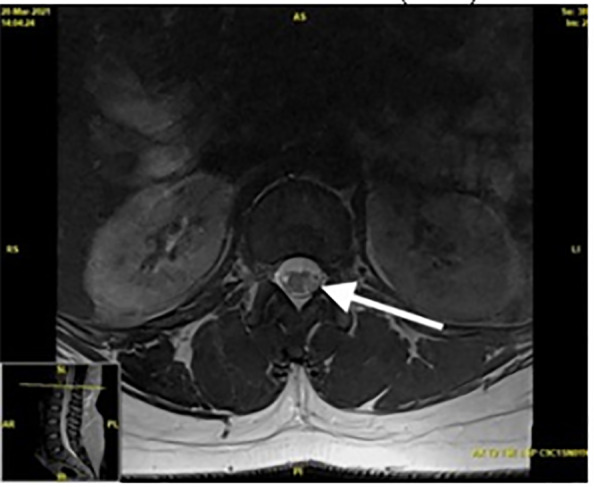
Axial T2-weighted magnetic resonance imaging showing a high signal intensity within the left spinal cord at the level of the first lumbar vertebra (arrow).
